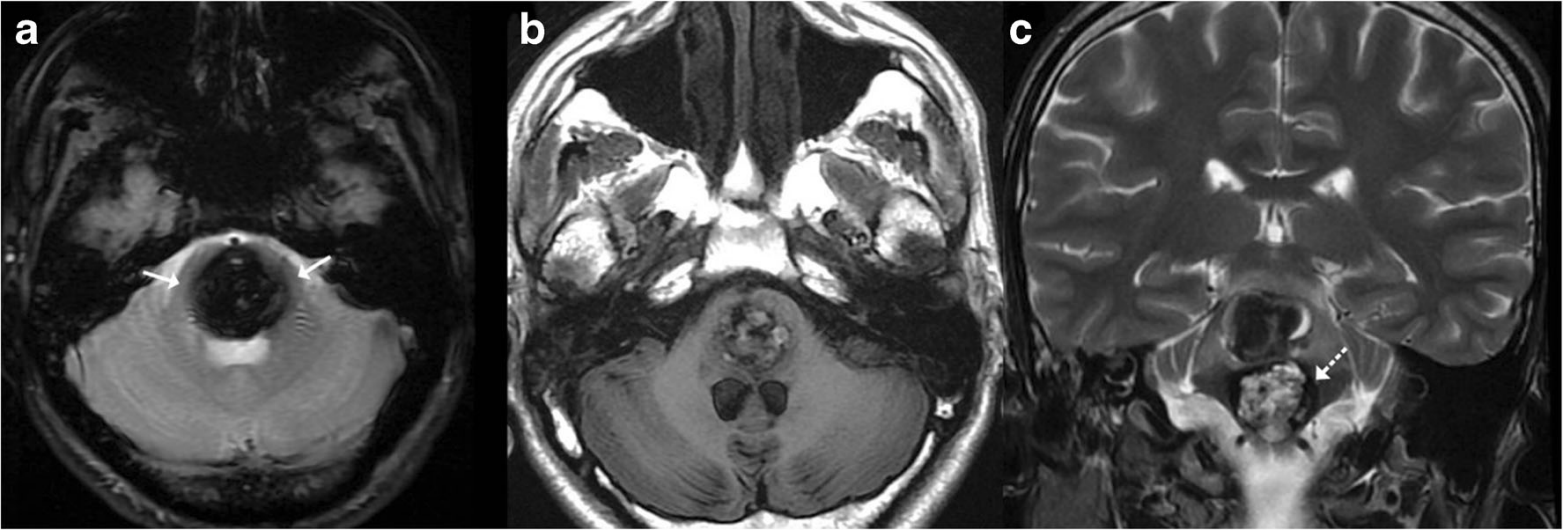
A 16-year-old patient with acute onset headache: a Susceptibility-weighted image shows prominent blooming in the pons (*arrow*). b T1-weighted images show heterogeneous areas of mixed hyper- and hypointensity consistent with blood degradation products giving a “popcorn appearance.” c Heterogeneous lesion with a prominent haemosiderin rim (*dotted arrow*) in keeping with cavernoma. There is an associated subacute haemorrhage in the superior aspect of the lesion
